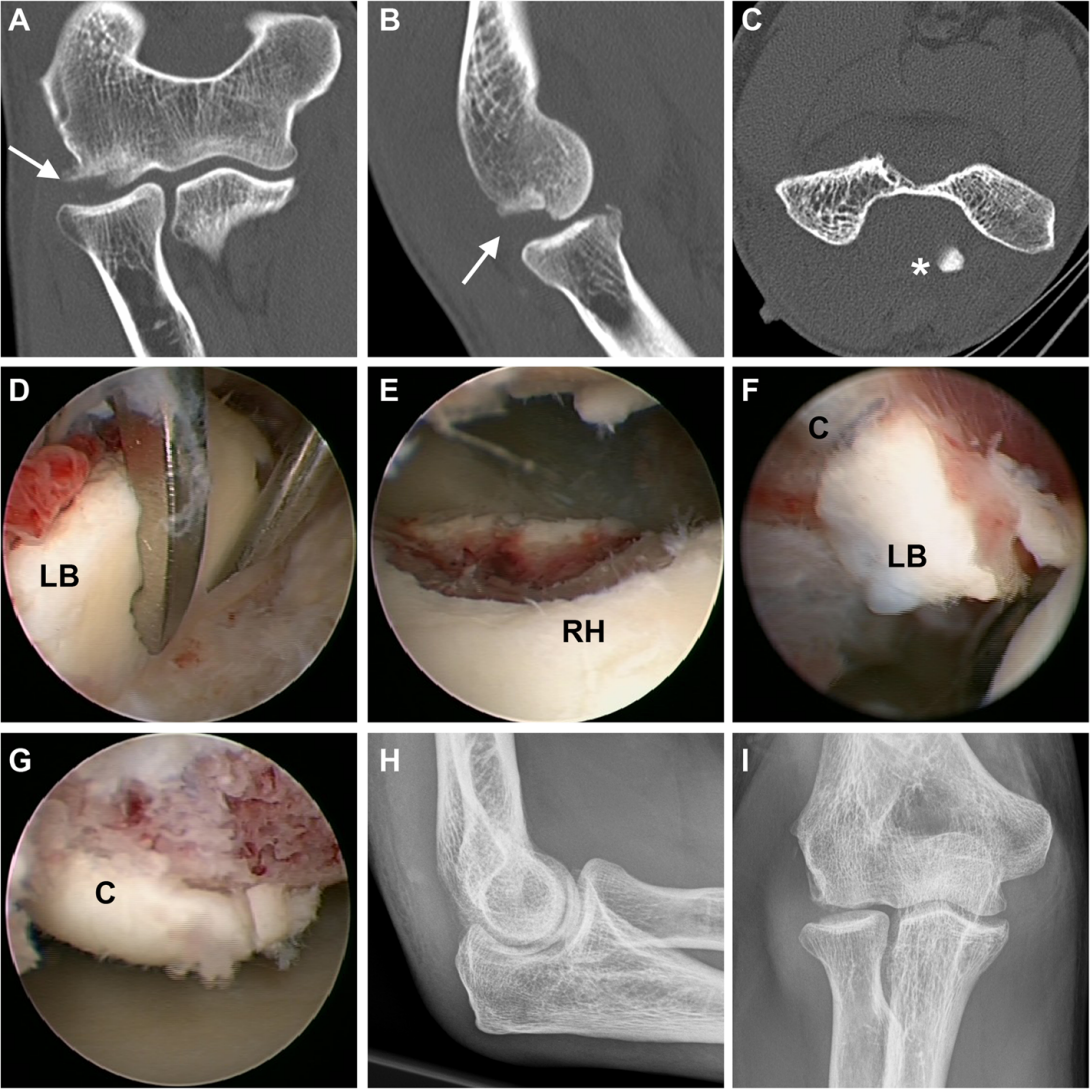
Radial head fracture classified as a type IV fracture due to an Osborne-Cotterill lesion [arrow] and displacement of the anterior rim fragment of the radial head into the fossa olecrani [*]. a-c CT scans. d Arthroscopic loose body [LB] removal. e Result after partial resection of unstable anterior radial head [RH] fragments. f A second loose body was found at the dorsal capitellum [C] near the (g) Osborne-Cotterill lesion. h, i Postoperative x-rays demonstrated correct alignment of the elbow joint and complete removal of loose bodies
